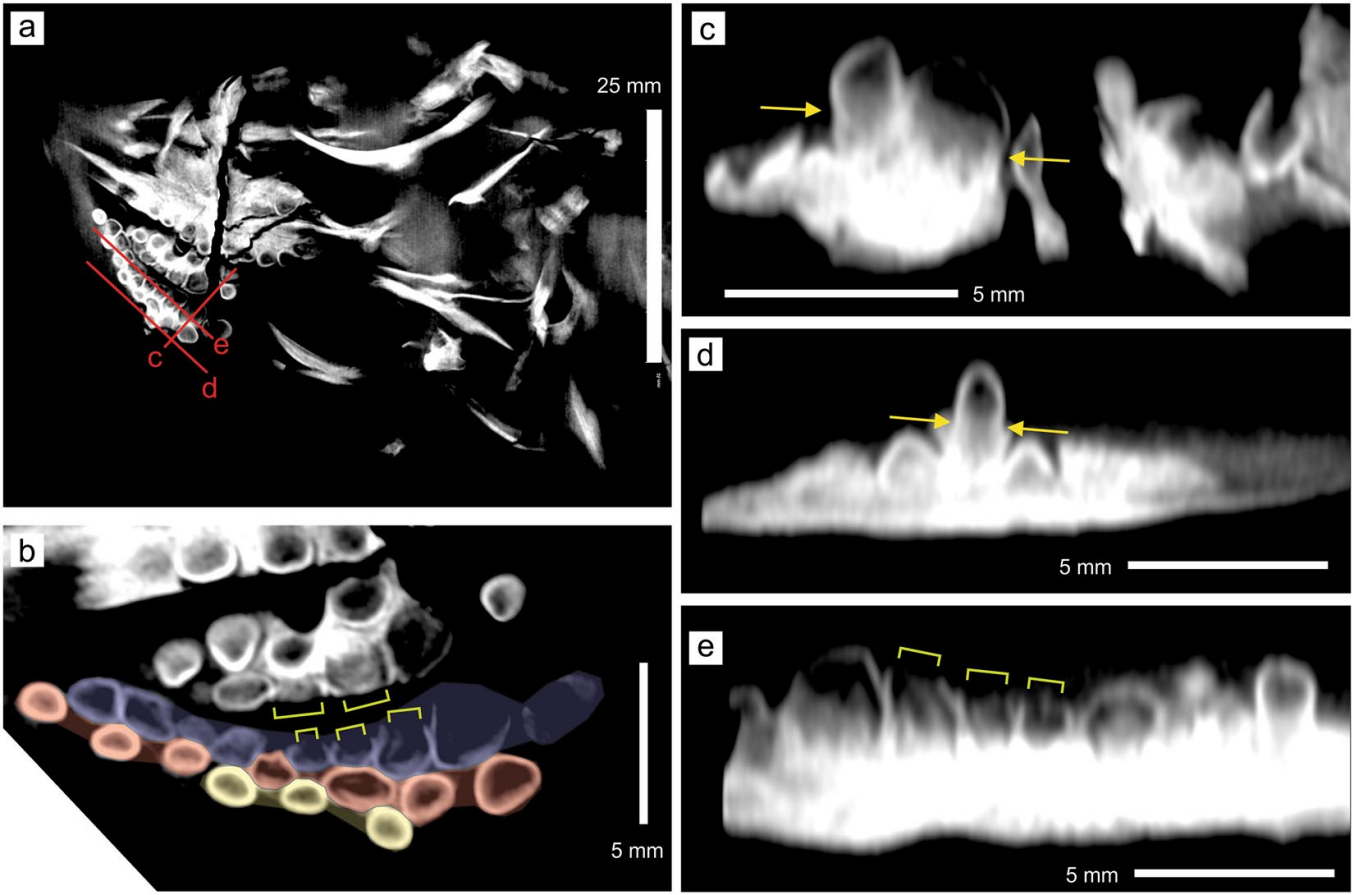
Sectional images of the 3D volume based on the CT images. (a) Section through the head region approximately parallel to the bedding plane. (b) A close-up view of the right dentary (below) and maxillary (middle to above) dentition in a, with a part of the left dentary teeth along the top margin. Dentary tooth rows are colored, with the labial row in blue, middle row in red, and lingual row in yellow. (c) A cross-section through the red line labeled c in a, nearly parallel to the longitudinal direction of the dentary teeth. (d) A cross-section through the red line labeled d in a, nearly parallel to the longitudinal direction of the dentary teeth. (e) A cross-section through the red line labeled e in a, nearly parallel to the longitudinal direction of the dentary teeth. Yellow allows point to the constriction between the crown and root. Yellow square brackets indicate the parts of tooth crowns without the enamel due to tooth wear.
